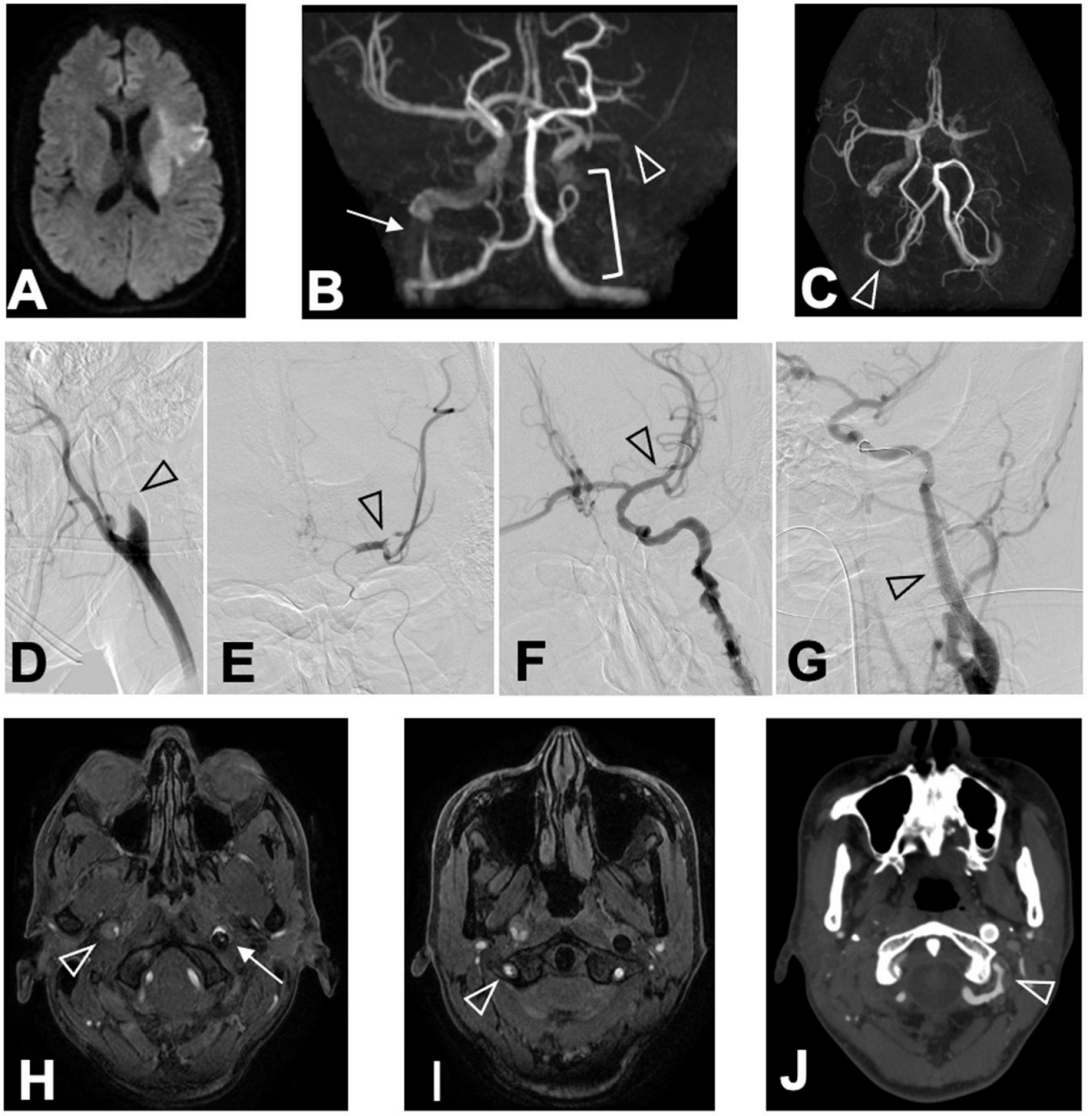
Chronological changes of CCAD lesions. MRI (DWI) on arrival revealed (A) hyperintense lesions in the left frontal lobe, the left temporal lobe, and the left corona radiata in DWI sequence. MRA revealed (B) M2 segment occlusion of the left MCA (arrowhead), occlusion of the left ICA (bracket), and severe stenosis of the right ICA (arrow) in coronal view, and (C) luminal irregularity of the V3 segment of the right VA (arrowhead) in basal view. Angiography conducted at ED revealed (D) the left ICA occlusion (arrowhead), (E) occlusion of the M2 segment of the left MCA (arrowhead), (F) recanalized left MCA after thrombectomy (arrowhead), and (G) stented left ICA after angioplasty (arrowhead). MRA performed 24 h after hospitalization revealed (H) an intimal flap in the right ICA (arrowhead), the stented left ICA (arrow), and (I) intimal flap in the right VA (arrowhead). CTA performed 15 days after hospitalization revealed (J) luminal irregularity of the V3 segment of the left VA (arrowhead).
